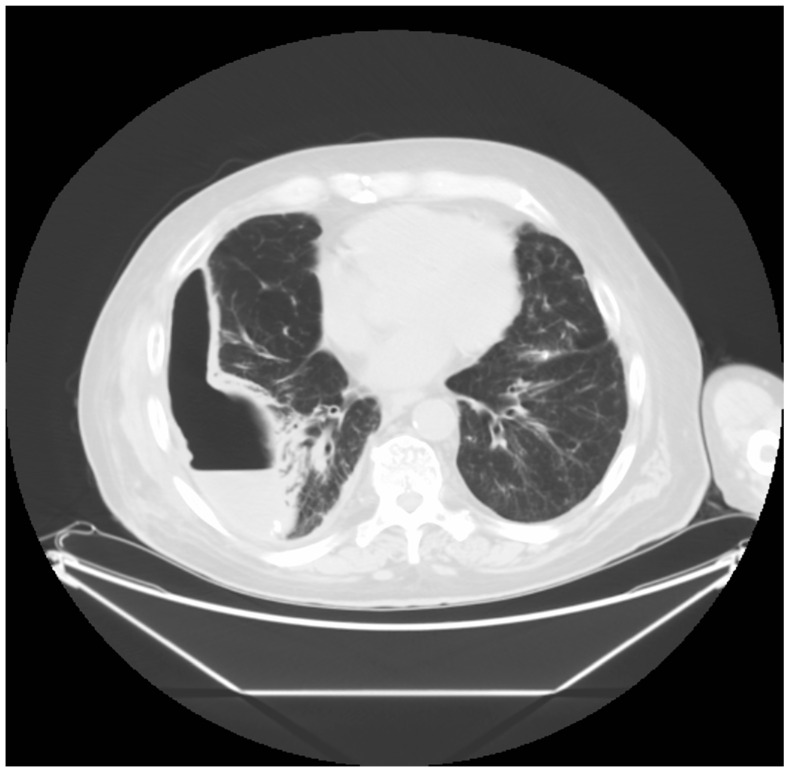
A CT scan image showing the trapped lung and free fluid, presumed to be a pyothorax or lung abscess. This condition was initially treated with guided percutaneous drainage.
